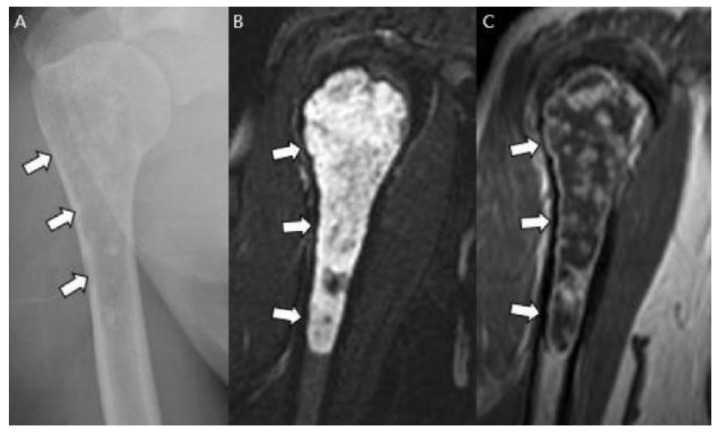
A typical cartilaginous tumor of the humerus in a 43-year-old woman. (A) Radiographs reveal a mixed lytic and sclerotic lesion in the humerus (arrows) with typical ring-and-arc calcifications. (B) Coronal T2-weighted image with fat suppression and (C) T1-weighted enhanced image demonstrate a lobulated chondroid tumor with longitudinal endosteal scalloping (arrows) along the 9 cm length of the tumor.
